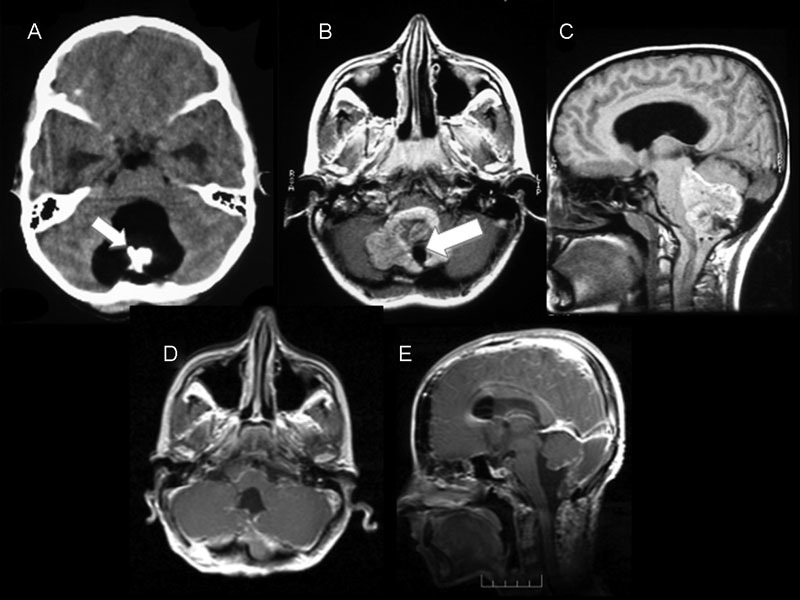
Preoperative and first postoperative radiologic studies of the patient. Axial computed tomography of the brain (A) showing a solid hypodense lesion situated in the fourth ventricle cavity containing a hyperdense bonelike area compared with the cerebral parenchyma in the center (small arrow). Magnetic resonance imaging (MRI) in (B) axial and (C) sagittal sections demonstrating a solid tumor hyperintense in T1-weighted imaging containing a hypointense core located in the fourth ventricle (large arrow). Postoperatively, (D) axial and (E) sagittal T1-weighted MRI with contrast medium showed the gross total resection of the lesion.
